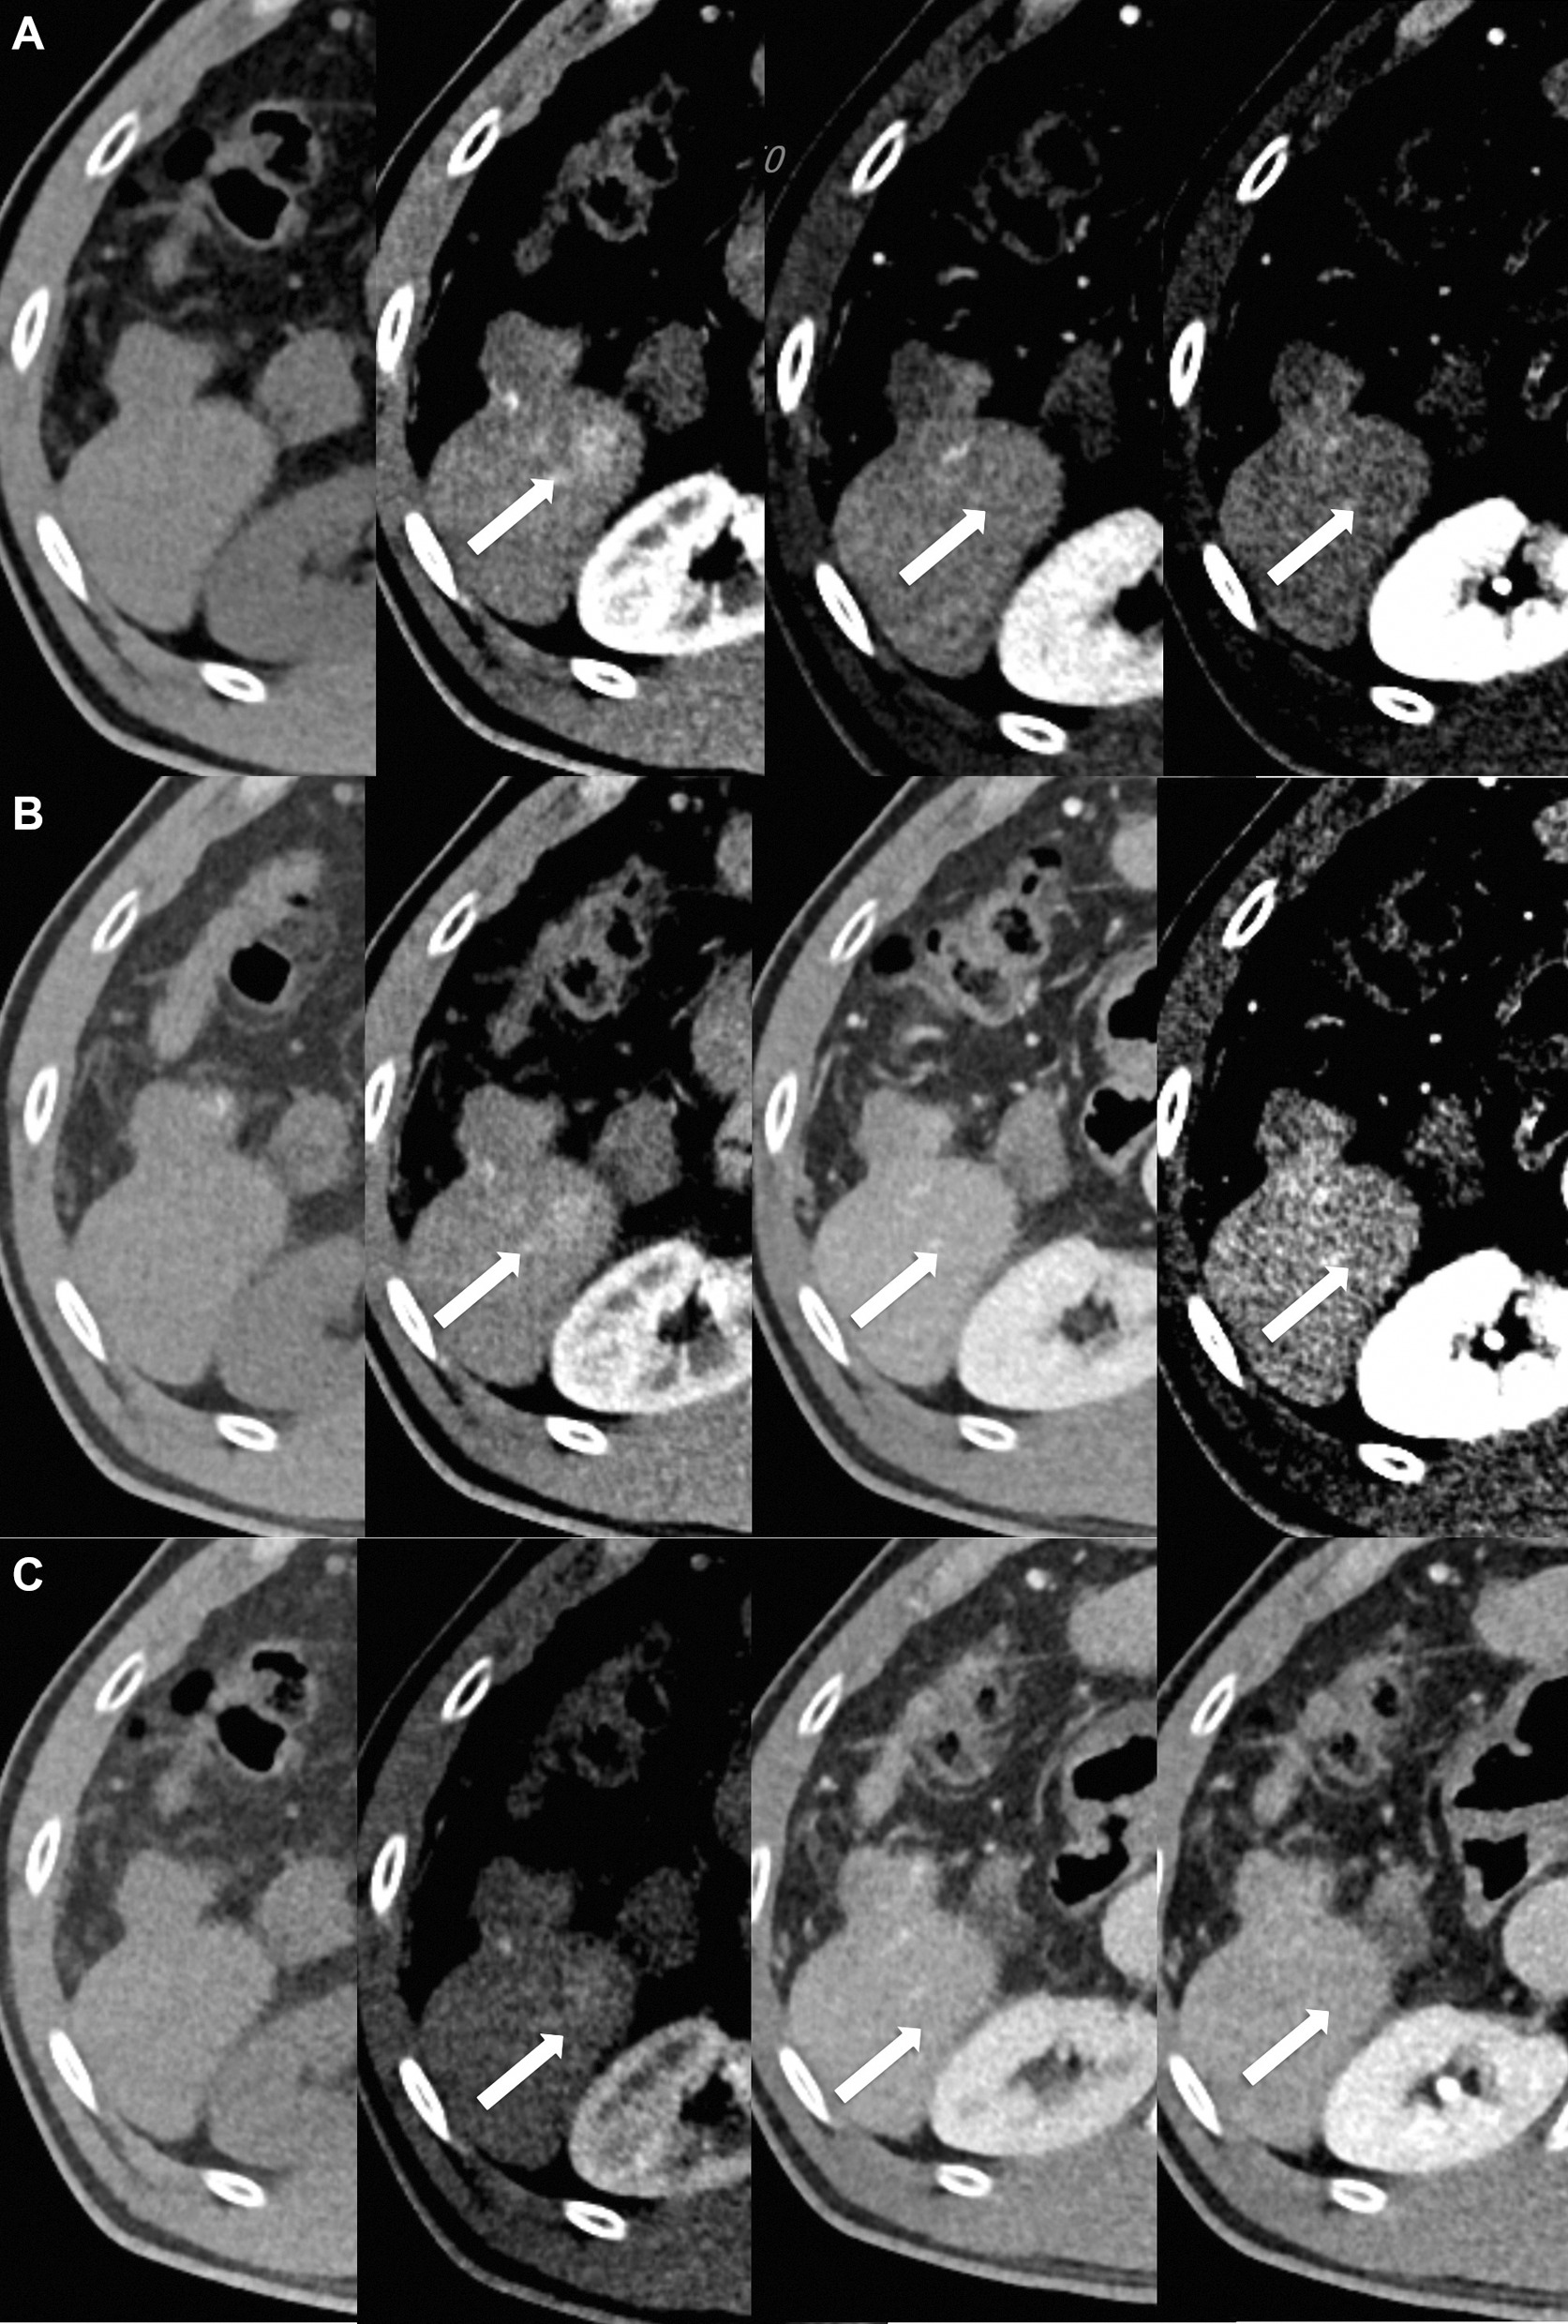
A 1.7-cm sized hepatocellular carcinoma in a 60-year-old man with 22.9 kg/m^2^ body mass index. A In standard-dose CT (9.3 mSv), reviewer 1 gave a LI-RADS score of 5, and reviewer 2 gave a score of 3. B In low-dose CT (6.5 mSv), both reviewers gave a LI-RADS score of 3. C In ultra-low-dose CT (2.8 mSv), both reviewers did not detect the lesion
